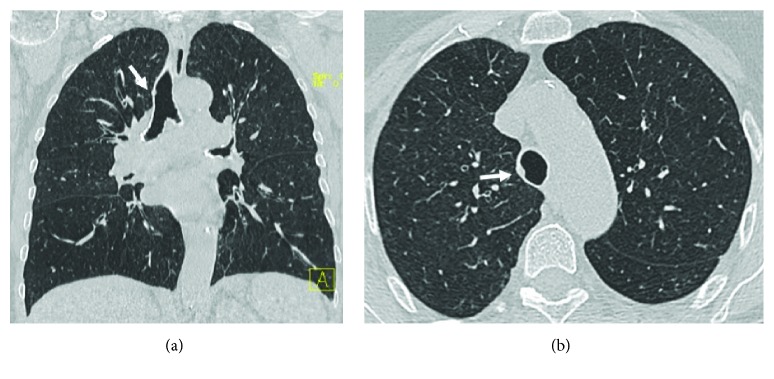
A 77-year-old woman with old history of CD. These HRCT coronal (a) and axial (b) reconstructions show a small nodule in the tracheal wall, most likely referring to a granuloma (arrows).
